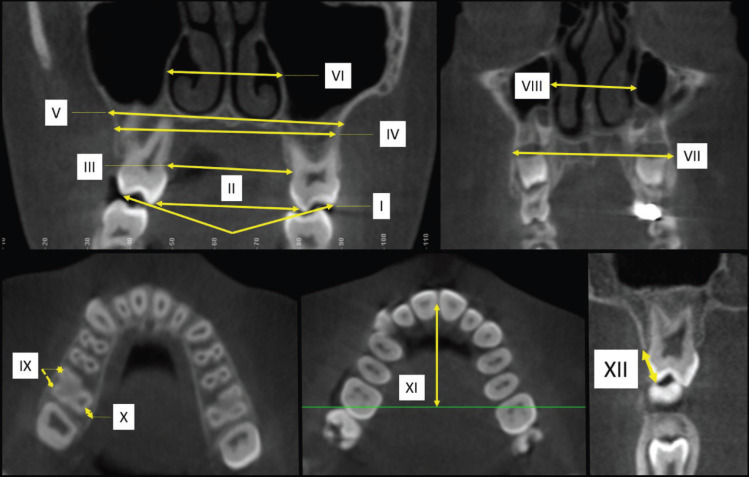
CBCT images showing the measurements of the different variables: A, Axial slices (posterior region): I - Tooth inclination, II - arch width (lingual) III - alveolar crest width (lingual), IV - maxillary width (external cortical), V – maxillary width (in the floor or the nasal cavity), VI - nasal cavity; B, Axial slices (anterior region): VII - Maxillary width, VIII - nasal cavity width; C, Coronal Slices (posterior region): IX - Thickness of the buccal (mesial and distal), X - thickness of the lingual bone plate; D, Coronal Slices (posterior region): XI - Arch perimeter; E, Parassagital images (posterior region): XII - level crest bone.
